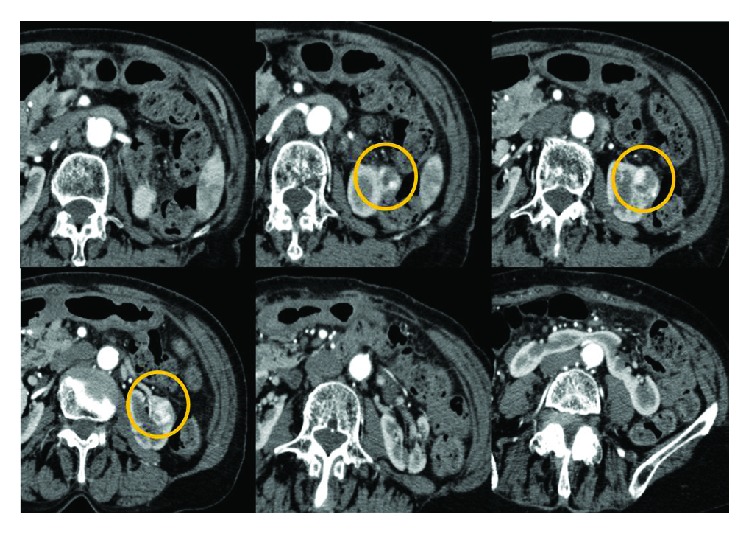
Contrast-enhanced CT. An enhanced tumor 20 mm in diameter was observed in the upper pole of the left-side horseshoe kidney.
